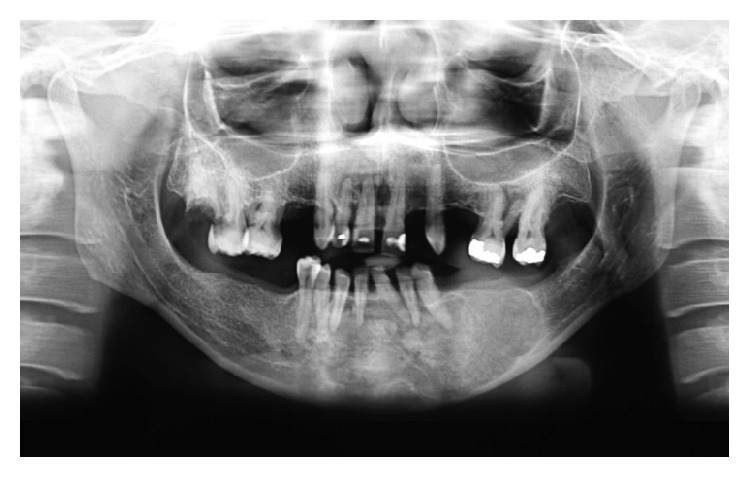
Panoramic radiograph indicates the left mandibular multilocular radiolucent lesion.
